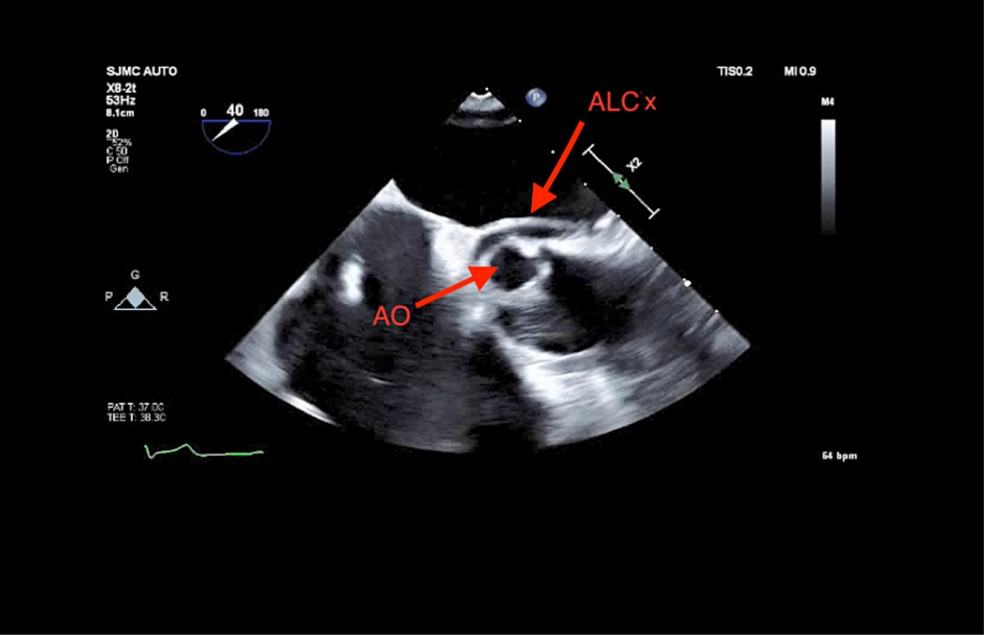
Anomalous left circumflex artery originating from right coronary artery transesophageal echocardiogram, short-axis view, AO= aortic valve, ALCx = anomalous left circumflex artery
